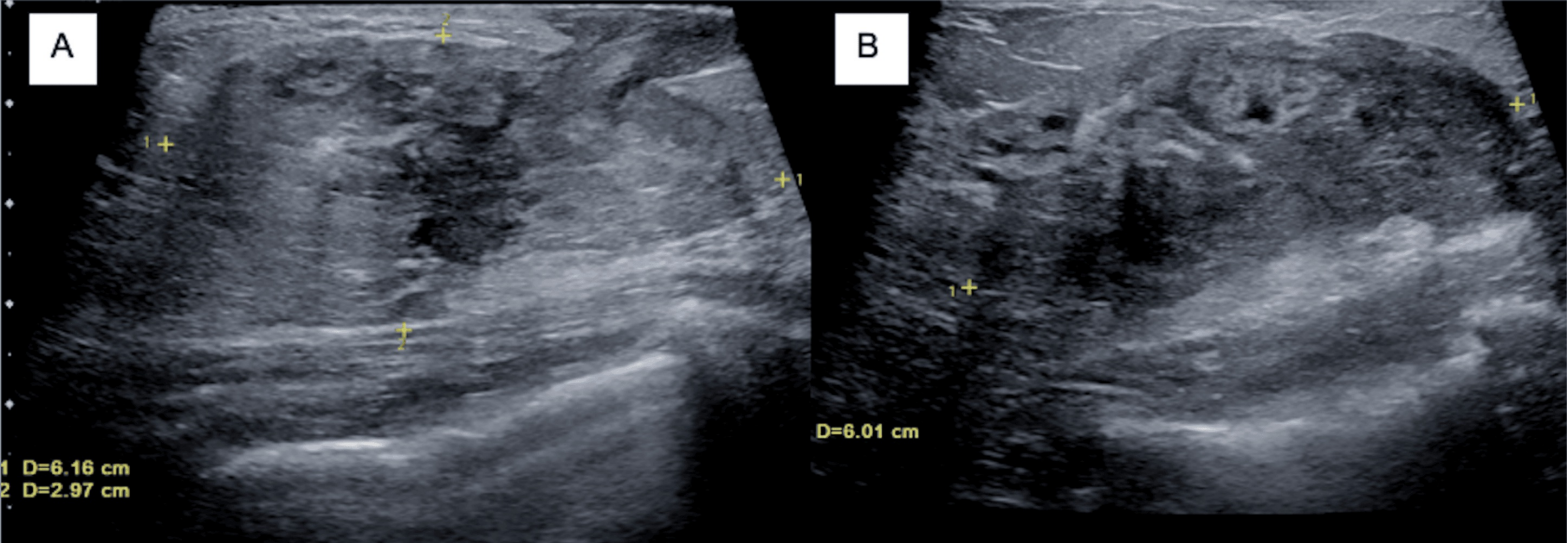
Ultrasound at initial visit: (A) antiradial and (B) radial view. Targeted ultrasound of the left breast at the area of palpable concern obtained at the patient’s initial evaluation demonstrated an ill-defined mixed solid and cystic lesion extending toward the dermis, corresponding to the site of clinical palpation.
